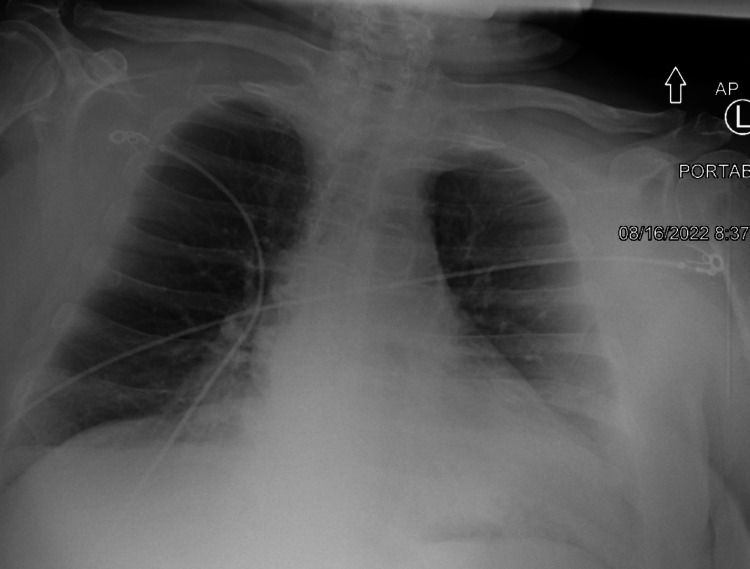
CXR showing borderline cardiomegaly. CXR: chest x-ray.
